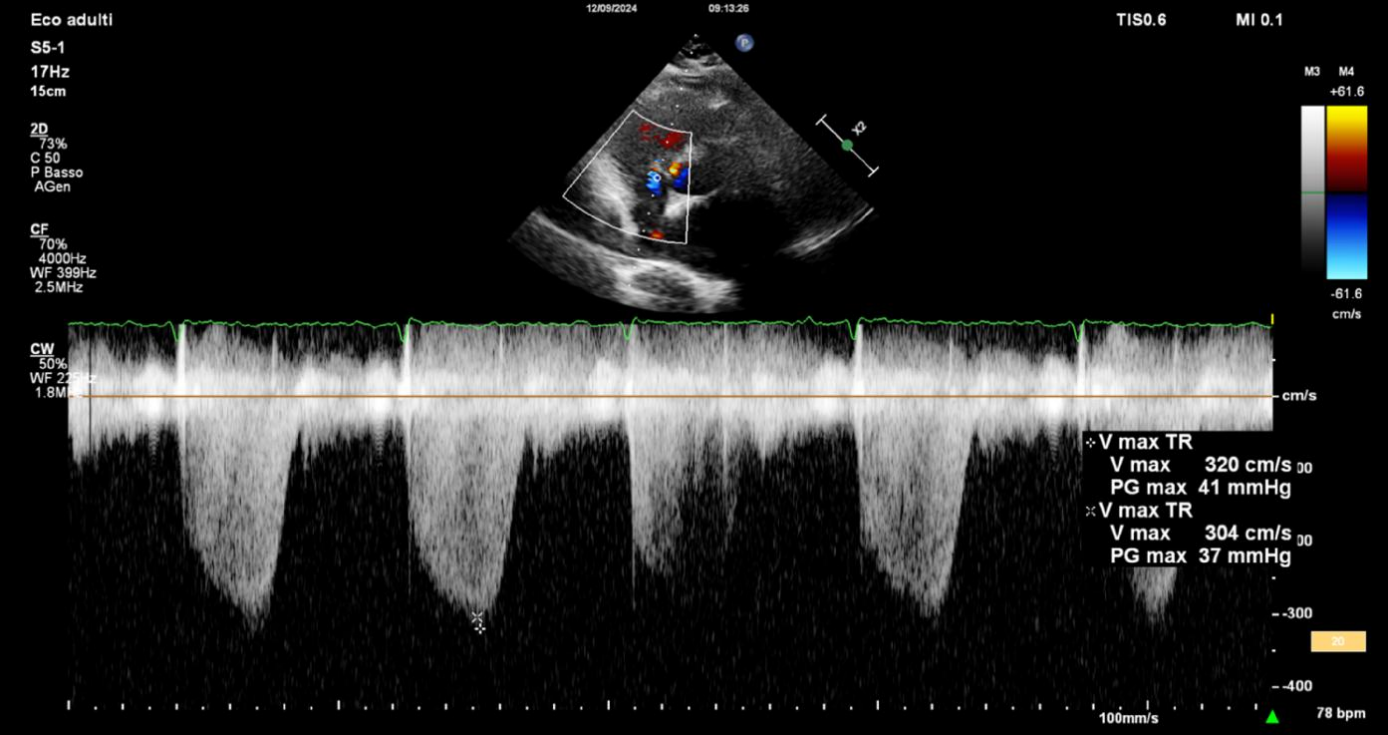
Doppler echocardiography showing a right ventricle-to-atrium pressure gradient of 37 mmHg and a regurgitation velocity greater than 2.8 m/s.
